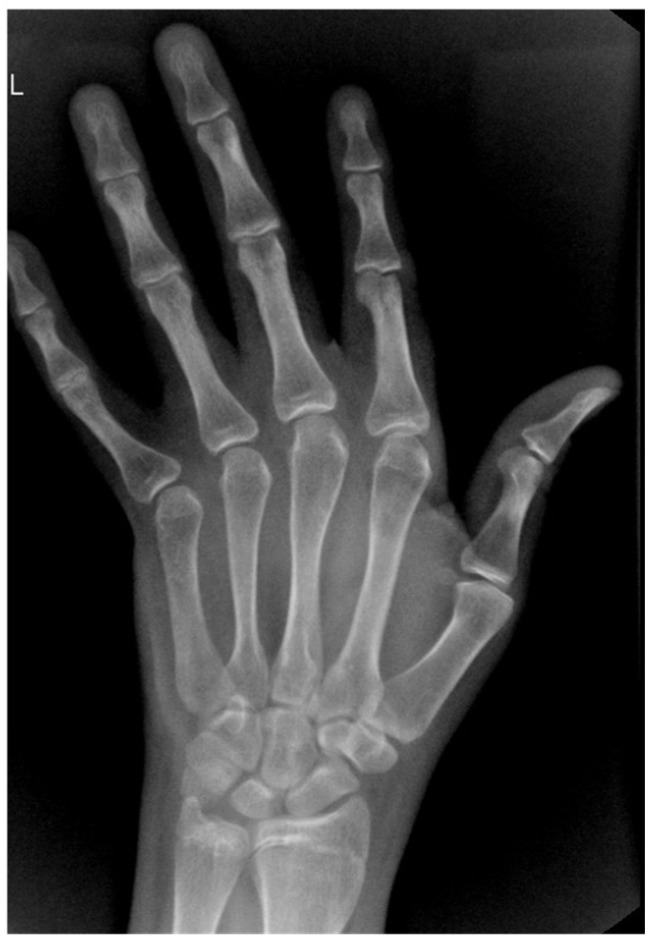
Case 1: left hand X-ray—bone age of 17 years.
